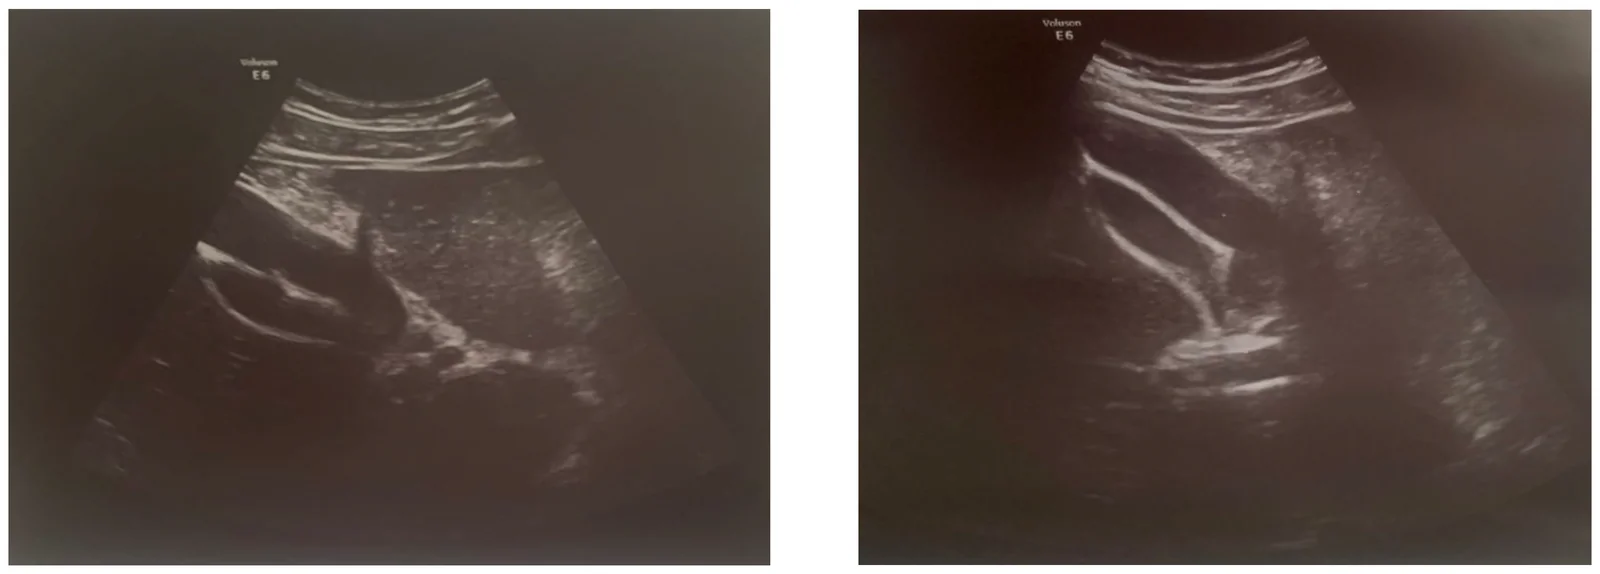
Transabdominal ultrasound demonstrating a duplicated, dual-chambered gallbladder without wall thickening or intraluminal cholelithiasis.
